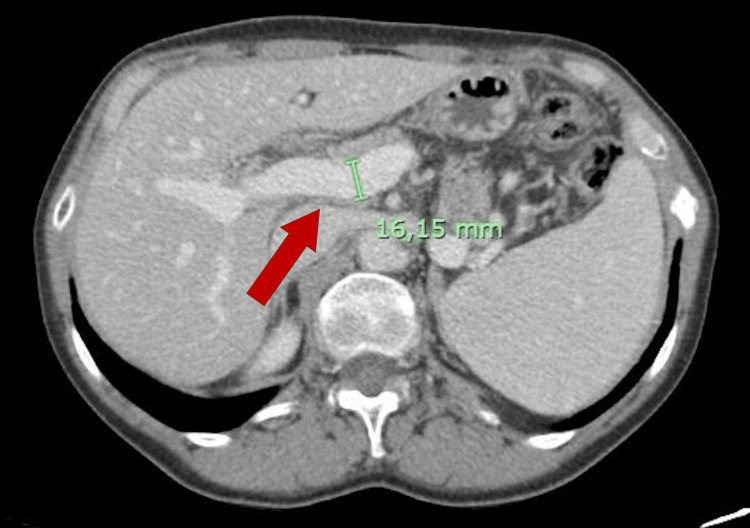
CT scan 19 months after T-DM1 treatment was started, showing de novo enlarged portal vein (red arrow).
